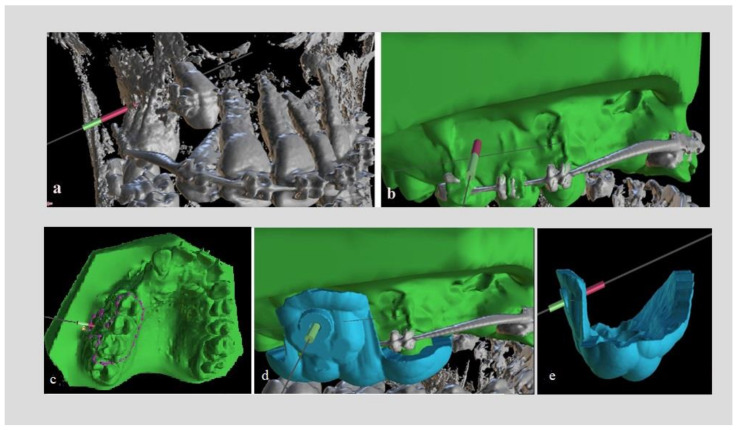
(a) Cone-Beam Computed Tomography (CBCT) image with virtual mini-implant in place. (b) Digital model A: Digital Imaging and Communications in Medicine (DICOM) and stereolithography (.stl) files combined. (c) Design of the surgical guide on the digital model. (d,e) .stl model of the surgical guide.
